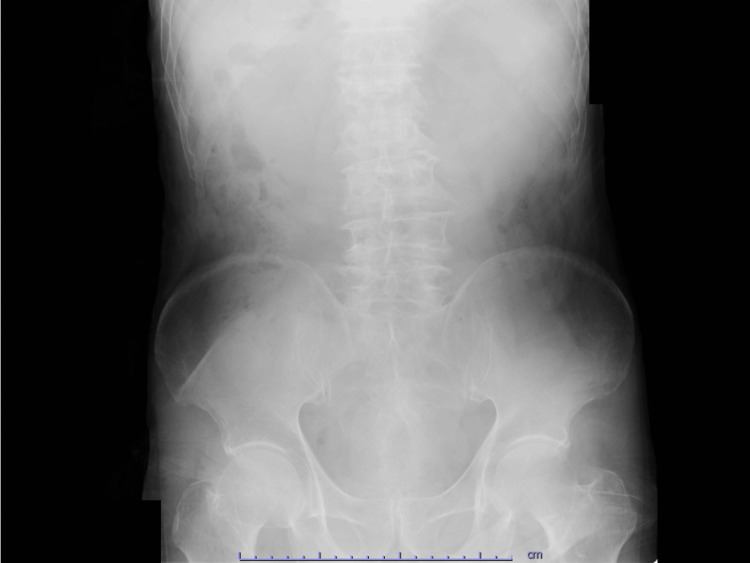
The abdominal X-ray on admission The abdominal X-ray on admission did not show signs of bowel obstruction, calcified density, and air in the biliary tract.
